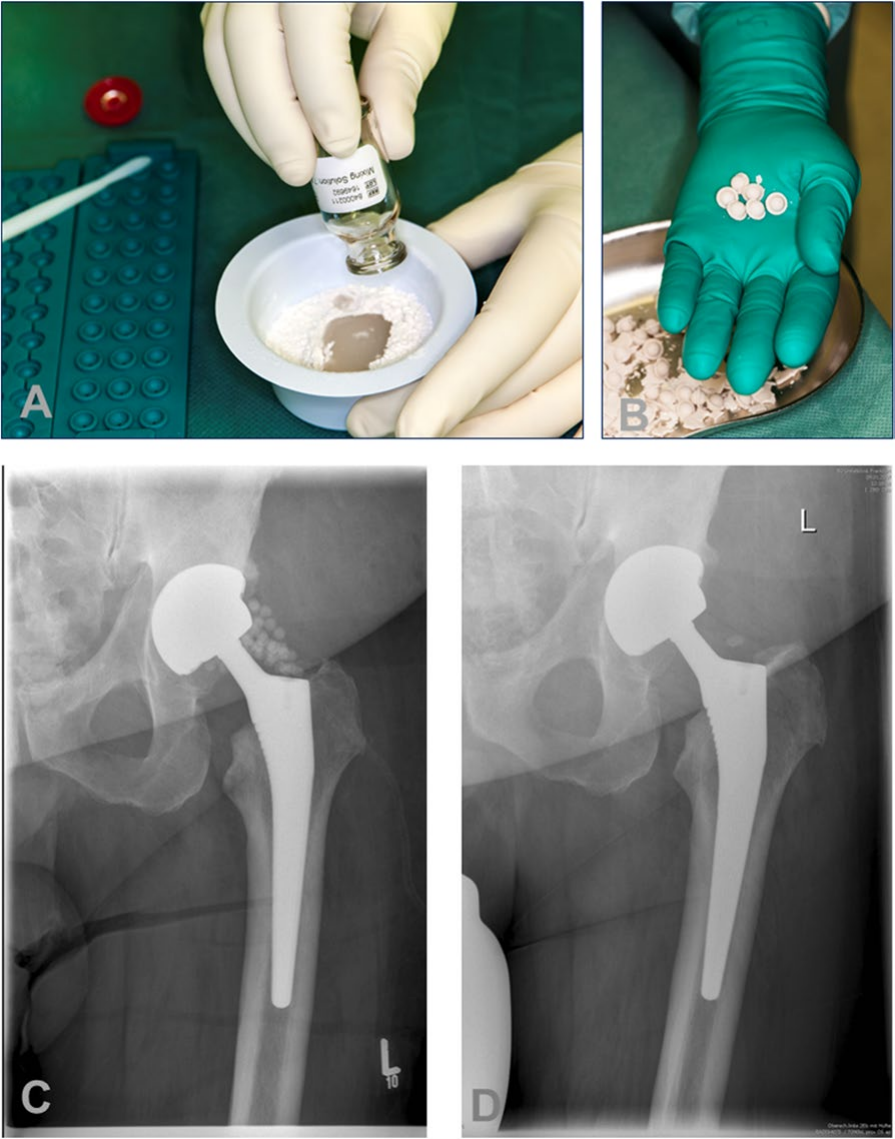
Bead application. a Application of the OSTEOSET (Wright Medical, USA) bead kit (admixed 3,000 mg of vancomycin per 30 beads). b, c Surgical single-stage application of beads in a periprosthetic joint infection of total hip arthroplasty during a debridement, systemic antibiotics, and implant retention (DAIR) procedure. d X-ray after one year shows degradation of the beads
